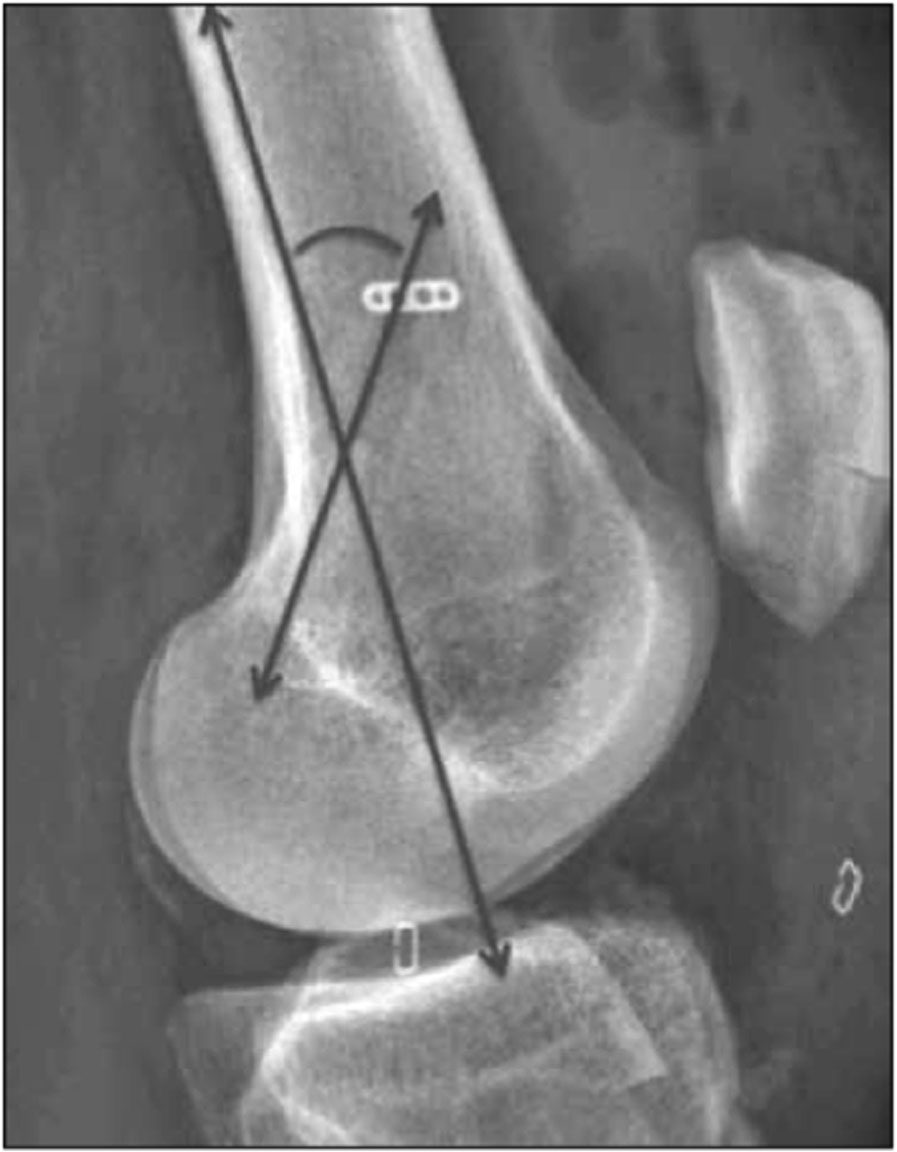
Measurement of femoral tunnel anteversion on true lateral postoperative radiographs. Anteversion is the angle between the posterior cortex of the femoral diaphysis and the line running long the middle of the femoral tunnel ending at the endobutton
